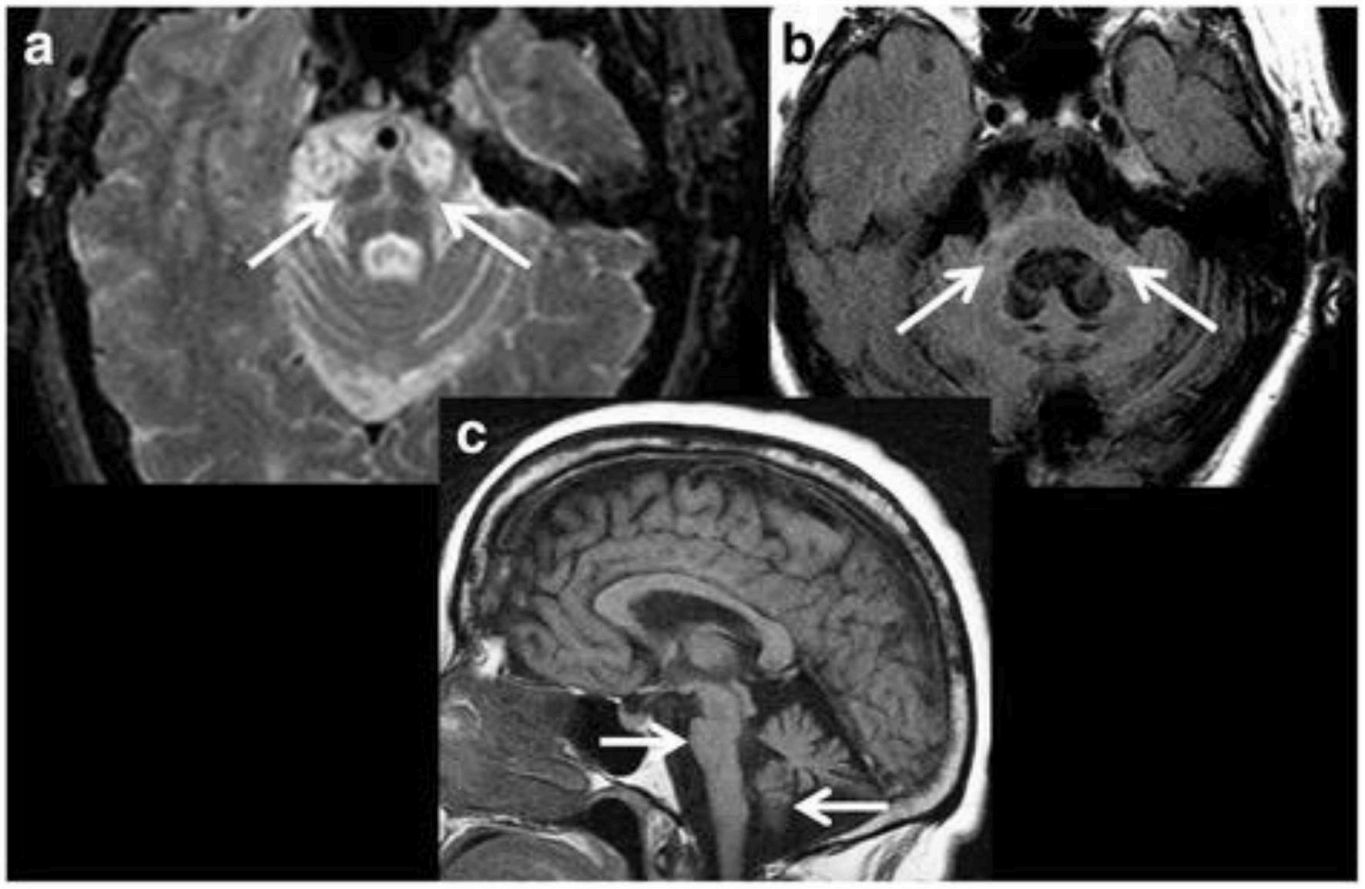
Magnetic resonance imaging of a patient clinically-diagnosed with multiple system atrophy (cerebellar type). (a) Axial proton density weighted sequence is presented at the level of pons, which shows cruciform pontine T2 hyperintensity as consistent with the “hot cross bun” sign, resulting from selective susceptibility of the pontocerebellar tract in multiple system atrophy (cerebellar type). In addition, disproportionate atrophy of the pons and partially visible cerebellar hemispheres are also apparent. (b) Axial fluid-attenuated inversion recovery (FLAIR) sequence is presented with cruciform T2 hyperintensity within the pons and middle cerebellar peduncles (i.e., “middle cerebellar peduncle” sign) along with marked atrophy. In addition, cerebellar hemispheric and vermian atrophy is evident with *ex vacuo* dilatation of the fourth ventricle. (c) Sagittal T1-weighted sequence is presented showing disproportionate atrophy of the brainstem and cerebellar vermis. Figure reproduced from Saeed et al. (10), under the Creative Commons Attribution License 4.0 (https://creativecommons.org/licenses/by/4.0/).
